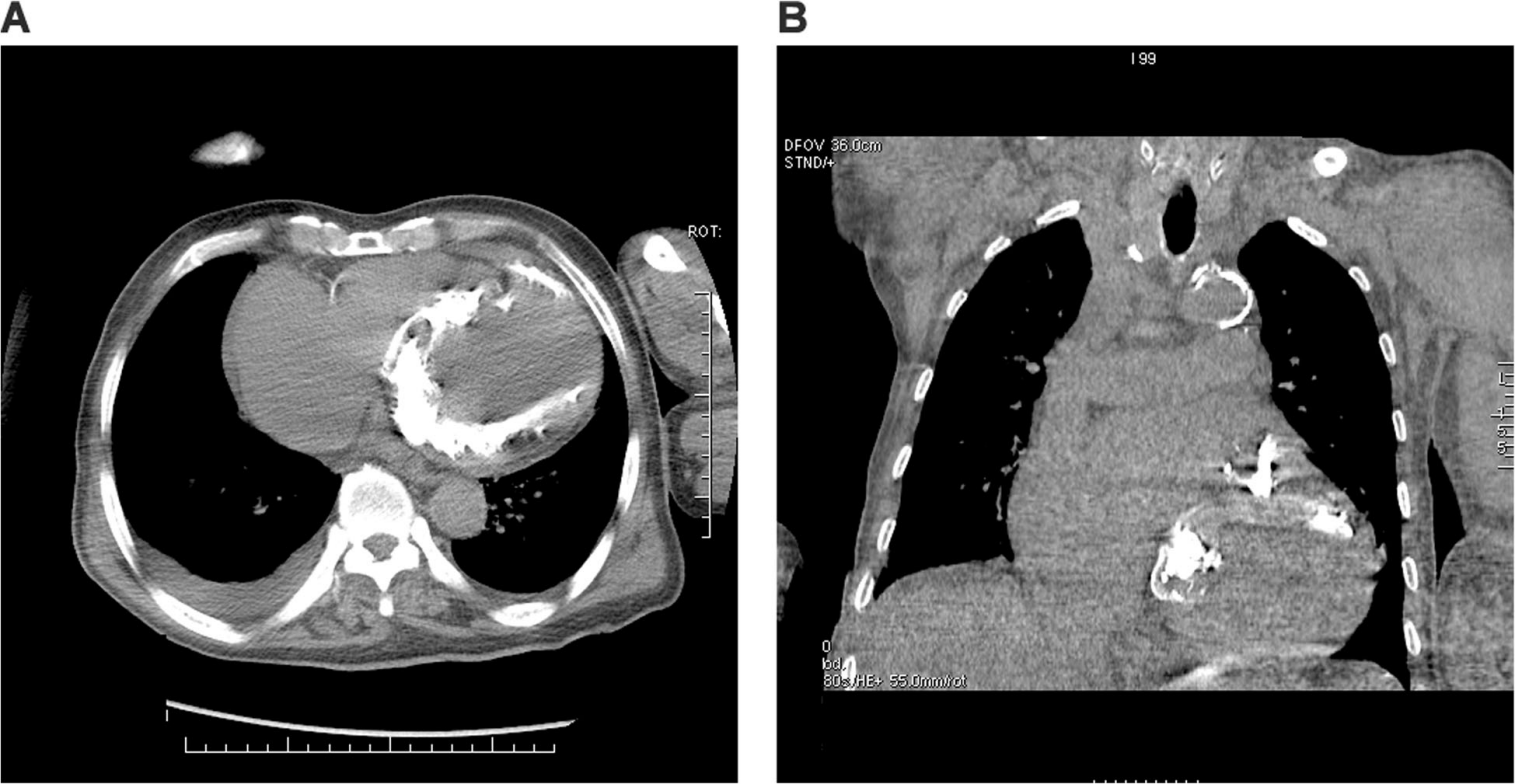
Computed tomography of chest, a Horizontal scan shows extensive calcification of left ventricular myocardium and interventricular septum. b Coronal scan with spots of patchy calcification on the left ventricle
